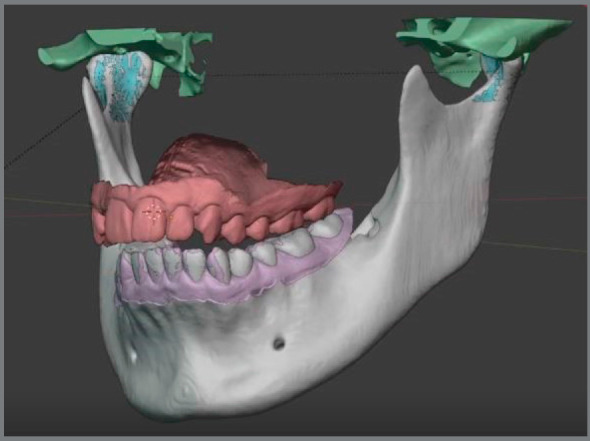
Virtual articulator based on segmented CBCT data, using Blender add-on ( Cavycon ).
